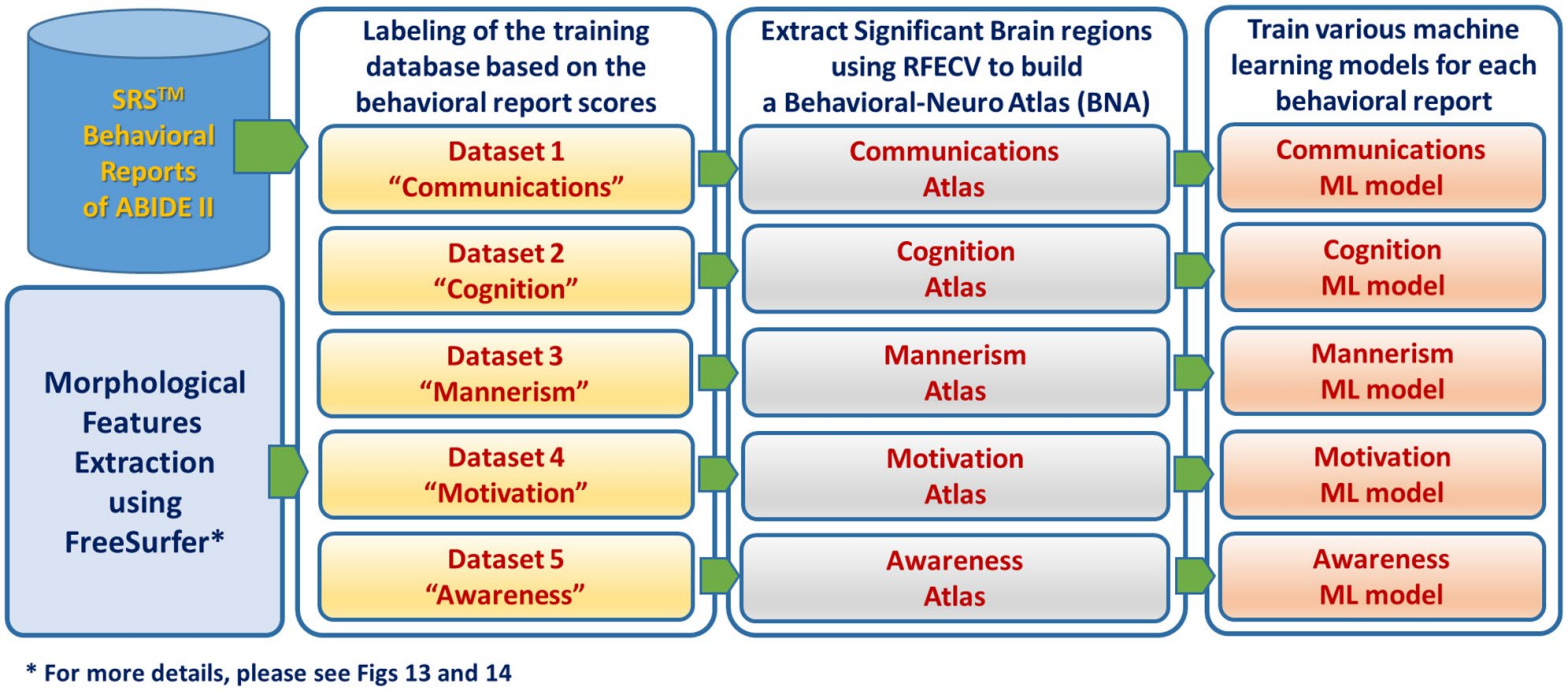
An outline of the proposed approach, starting from the acquisition of MRI volumes to the diagnosis. Note that the training of the ML models in the right panel involves hyperparameter optimization.
